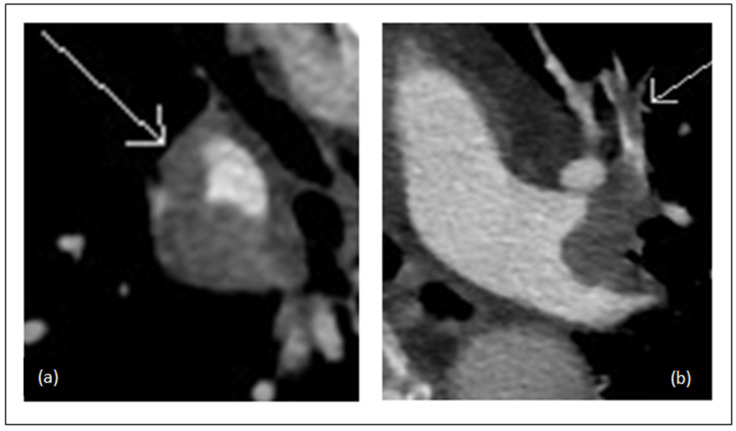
CT angiography in a patient with acute pulmonary embolism. (a) Partial occluding thrombus in right basal trunk (Modified Miller score = 4); (b) Partial occluding thrombus in the left apical anterior segmental artery (Modified Miller score = 1).
